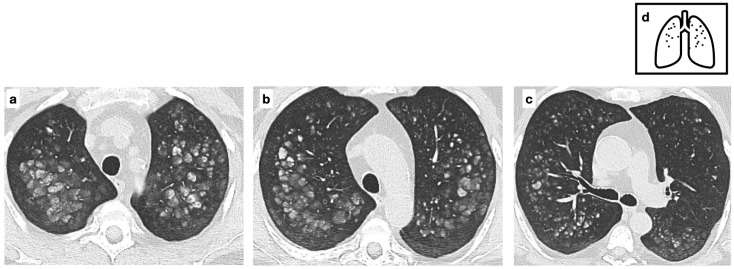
Metastatic pulmonary calcification due to deposition of excess calcium in a background of normal lung parenchyma (a–c). These nodules are typically located in the upper lobes. HRCT images show fluffy centrilobular ground glass opacities, some of which calcified. Pattern of calcification (d). (Curtesy of E. Bezzon).
